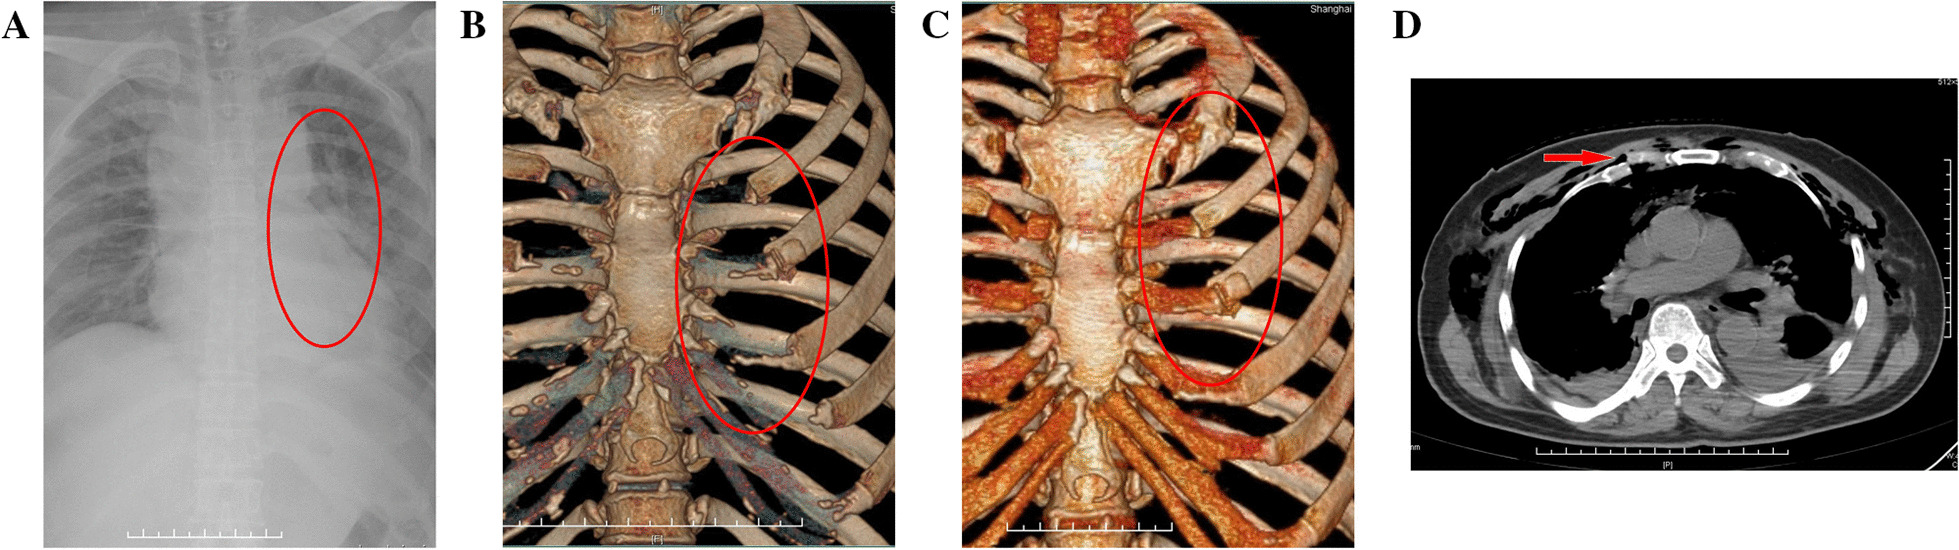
Showing an x-ray film and two kinds of CT images of costal cartilage fractures from the same patient. A Showing costal cartilage fractures are almost impossible to show on normal X-ray. B Showing costal cartilage fractures are occult on normal 3D CT image. C, D Showing costal cartilage fractures clearly on transverse section of CT image and cartilage specified 3D reconstruction CT image
